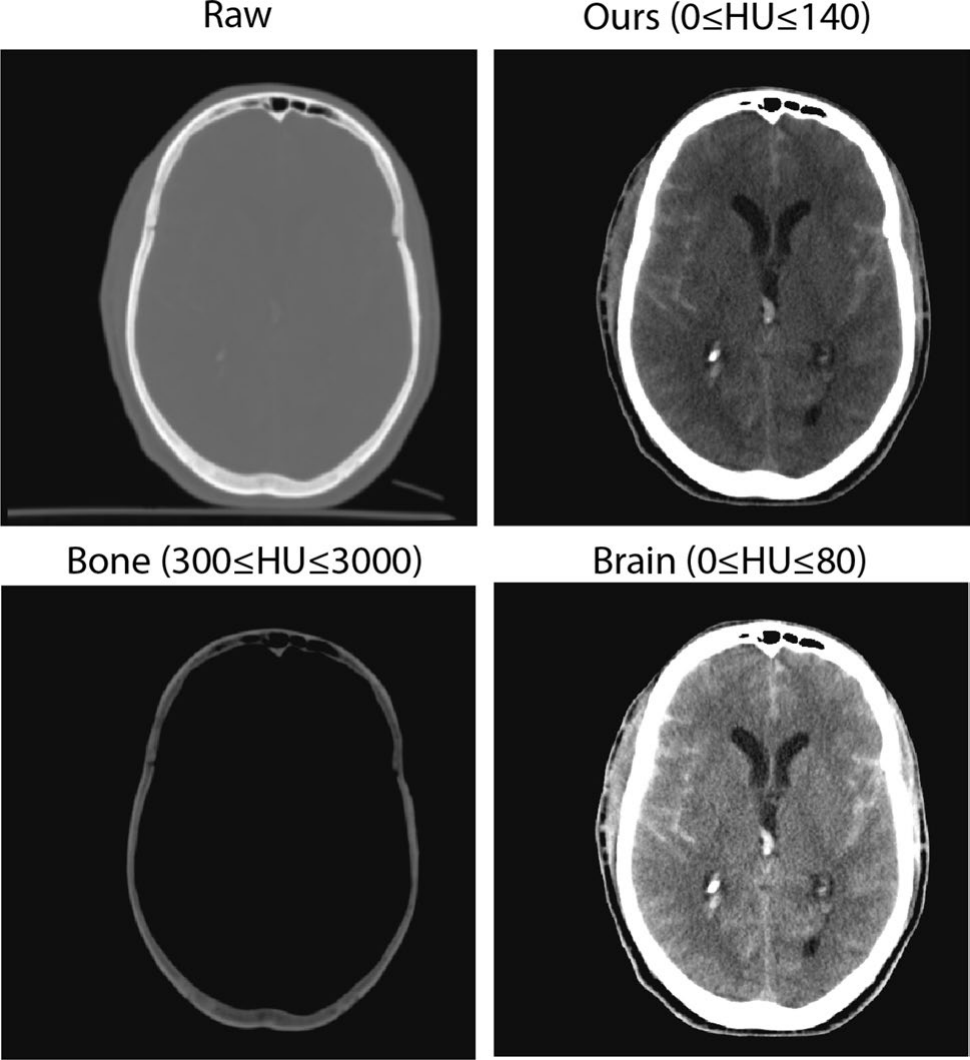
Contrast adjustment of CT scans with different window width settings
